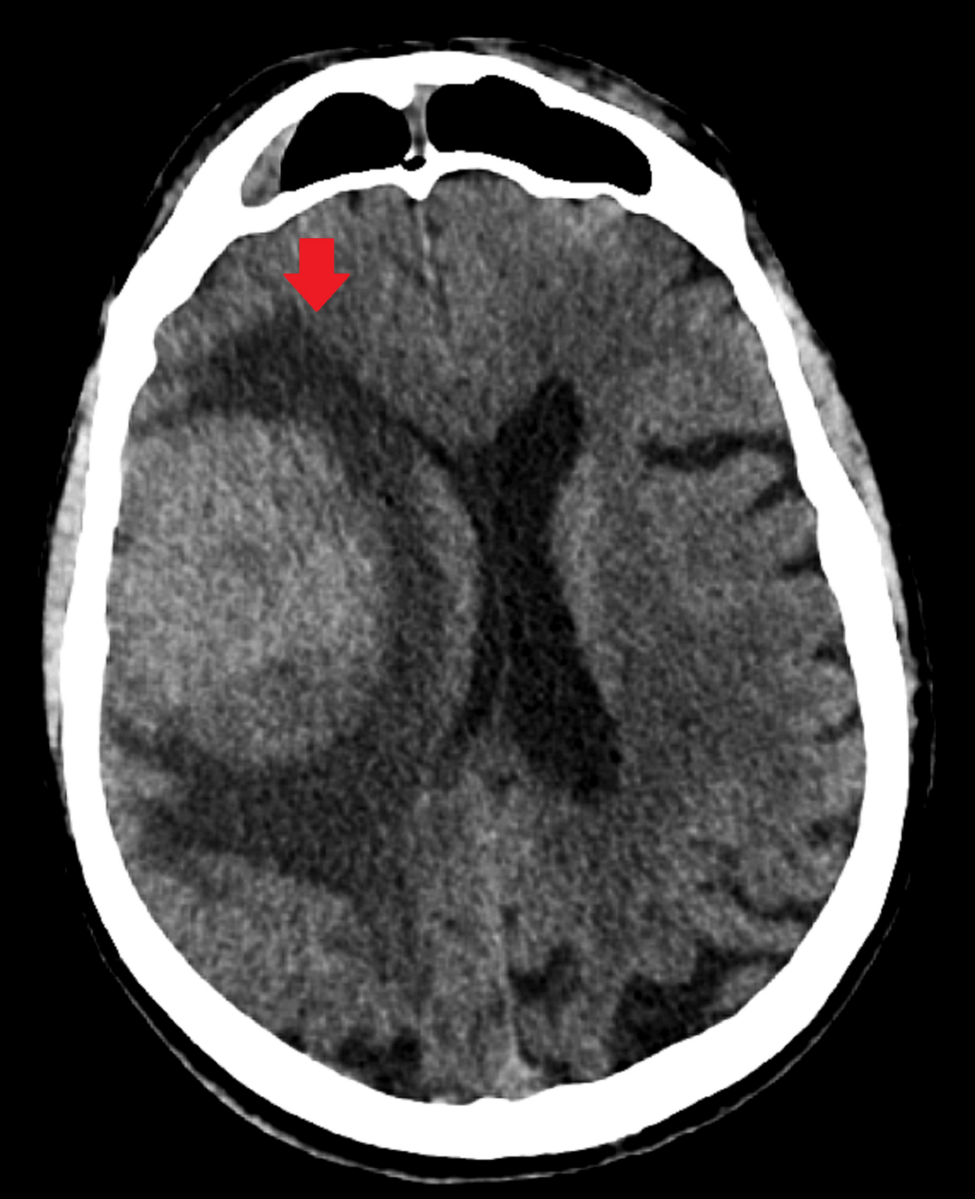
Transverse section of CT of the brain shows a brain mass
